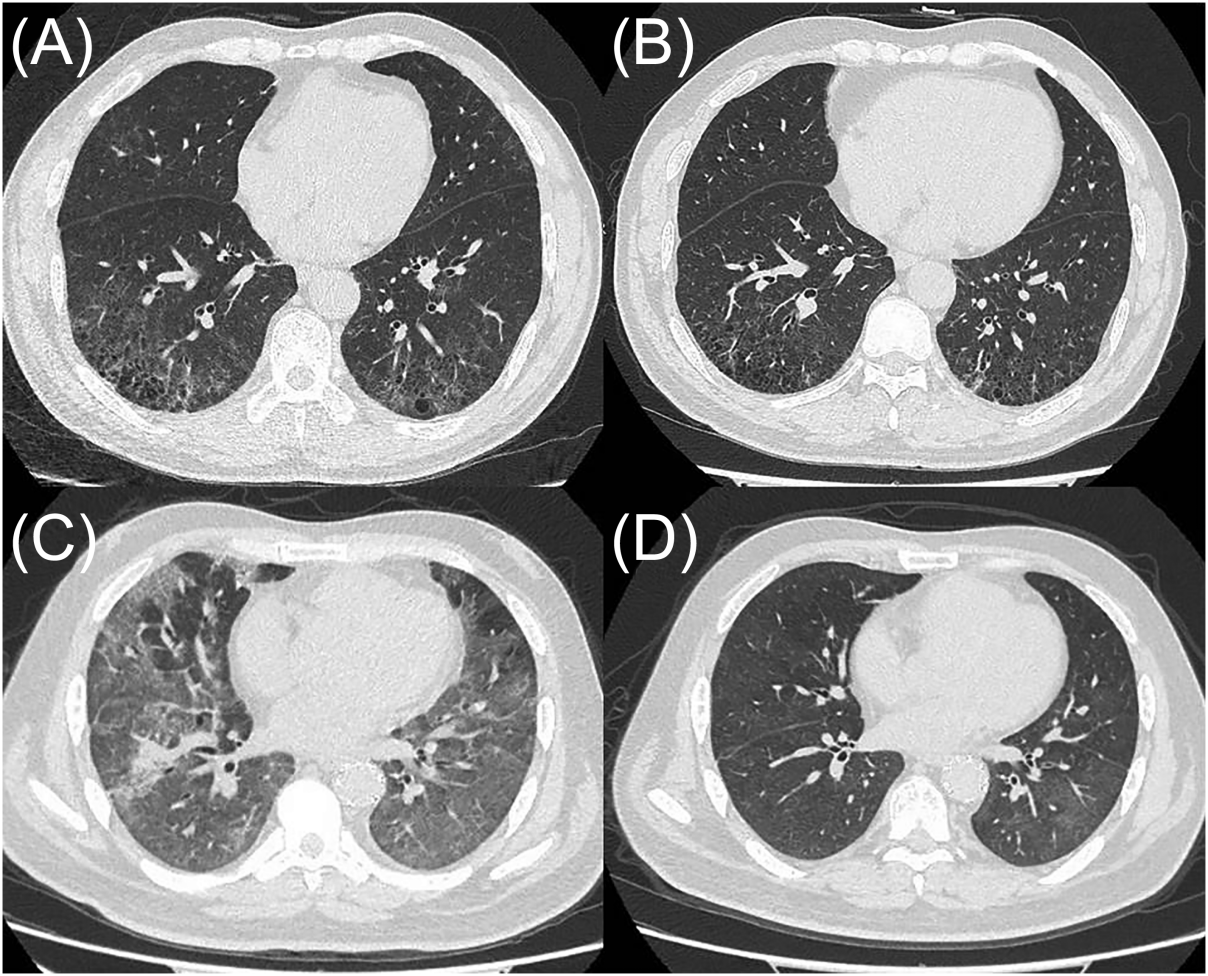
High‐resolution computed tomography (CT) of the chest: (A, C) Images of the two patients showed different degrees of diffuse ground glass opacity and traction bronchiectasis before treatment (B, D) the ground glass opacities decrease and traction bronchiectasis regressed obviously after treatment
